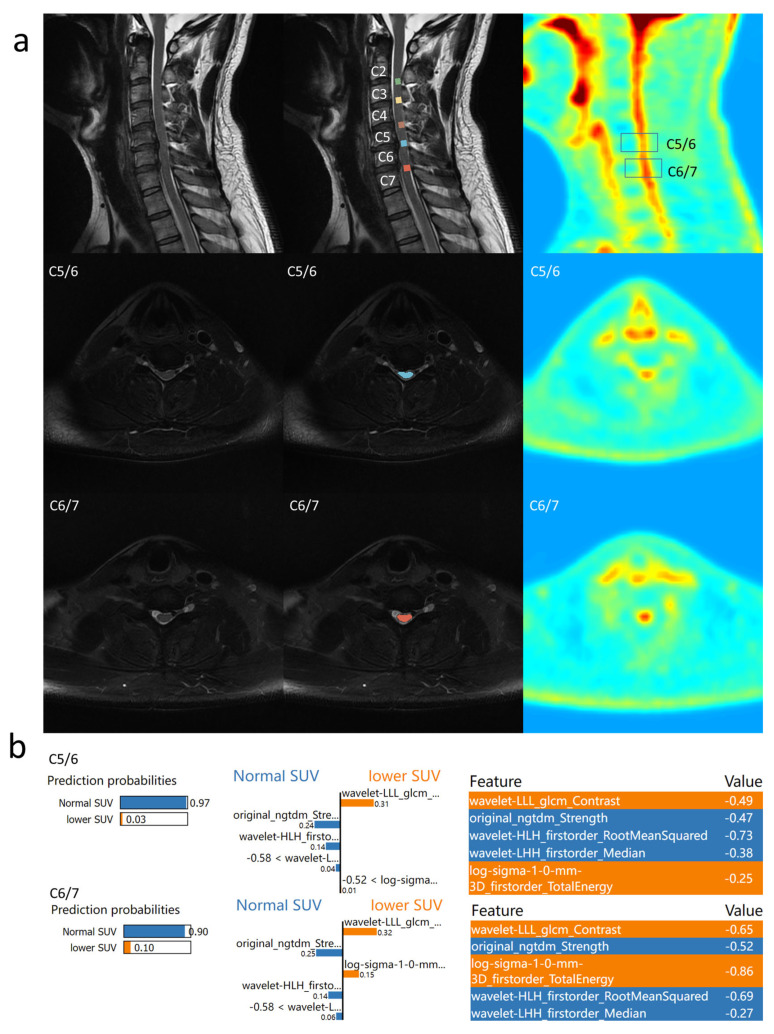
Prediction result of Example 2: (a) the PET/MR results of Example 2 are shown. Cervical spinal cord segments from C2 to C7 and five intervertebral disc segments were labeled. SUV_max_ decreased at the C5/6 level and SUV_max_ was normal at the C6/7 level; (b) the LIME results of the proposed model are shown. The proposed methods predicted that both SUV_max_ were normal at the two levels.
